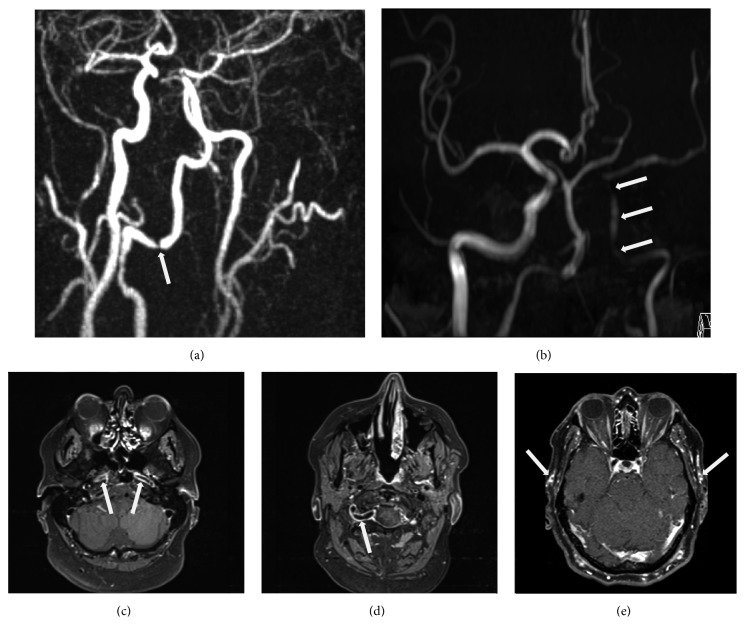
Magnetic resonance images of the affected arteries. High-grade stenosis of the right vertebral and the left distal internal carotid artery ((a), arrow) on a contrast enhanced magnetic resonance angiography. Long stenosis of the left ICA with tapering aspect ((b), arrows) on a follow-up time of flight MRI. Vessel wall enhancement of both internal carotid arteries ((c), arrows), and the right vertebral artery ((d), arrow), visible on a “dark blood” sequence. Contrast enhancement of both superficial temporal arteries ((e), arrows).
